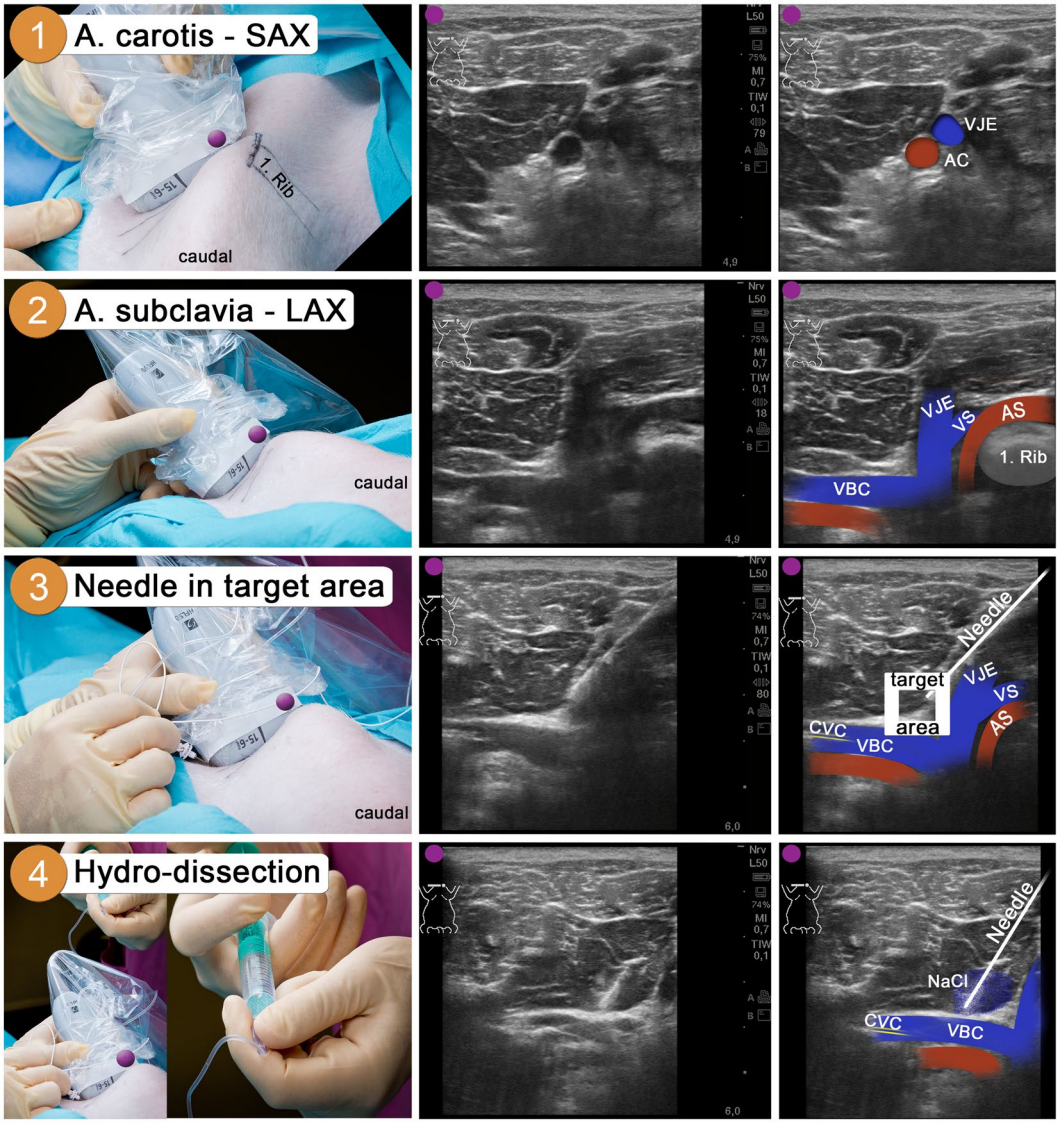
4-step approach for needle placement in the target area on the right side. Left: linear probe position; Middle: corresponding B-mode sonogram; Right: identical sonogram including sonoanatomic labeling. The purple dot indicates orientation of the sonographic probe. Step 1 (picture): Linear probe right above 1. Rib; Step 1 (sonogram): Transversal view on the carotid artery (AC) and the external jugular vein (VJE) in short-axis view (SAX). Step 2 (picture): Tilting probe thoracal to visualize landmarks; Step 2 (sonogram): Oblique view on subclavian artery (AS) and vein (VS) crossing 1. Rib in long-axis view (LAX), confluence of VS with VJE and left brachiocephalic vein (VBC). Step 3 (picture): Needle insertion under aspiration; Step 3 (sonogram): In-plane positioning of the needle in target area (white square). Step 4 (picture): Sodium chloride bolus; Step 4 (sonogram): Hydro-dissection of the phrenic nerve. CVC: Central venous catheter, NaCl: Sodium chloride.
